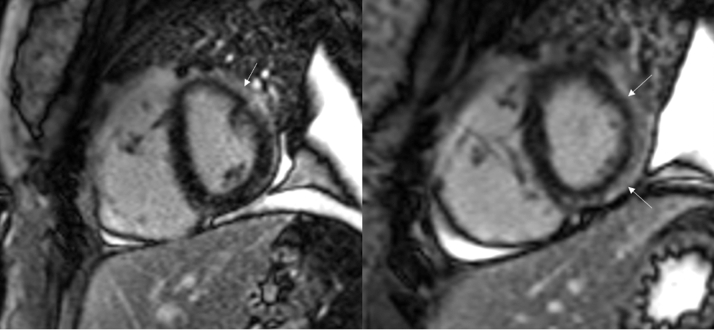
Short-Axis Delayed Enhancement Cardiac MRI Through the Midcavity The images demonstrate diffuse subepicardial late gadolinium enhancement of the anterolateral and inferolateral left ventricular myocardium as well as the adjacent pericardium. Note the D-shaped configuration of the left ventricle with interventricular septal flattening in the setting of right ventricular overload. The arrows indicated the areas of late gadolinium enhancement. MRI = magnetic resonance imaging.
